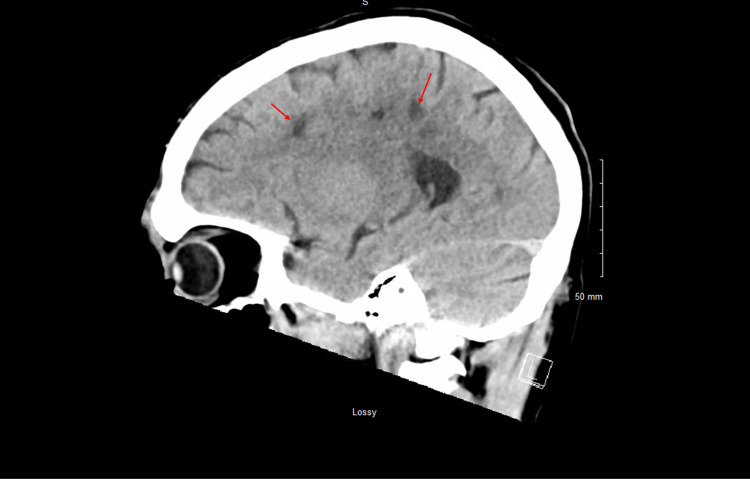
CT head sagittal view plain revealed multiple white matter hypodensities concerning septic emboli (Red arrows).
